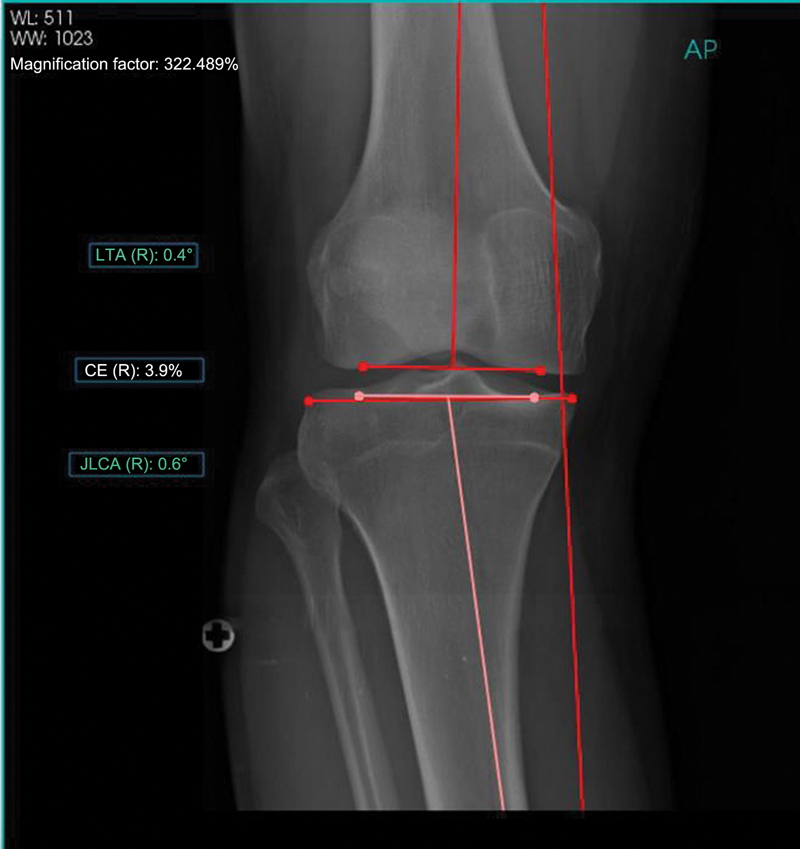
Anteroposterior knee radiograph with angular measurements.
